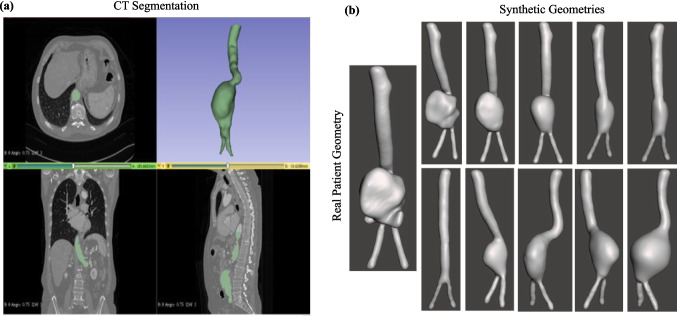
a 3D Geometry extraction from CT images. b 10 synthetic geometry generation from a single real patient’s geometry
